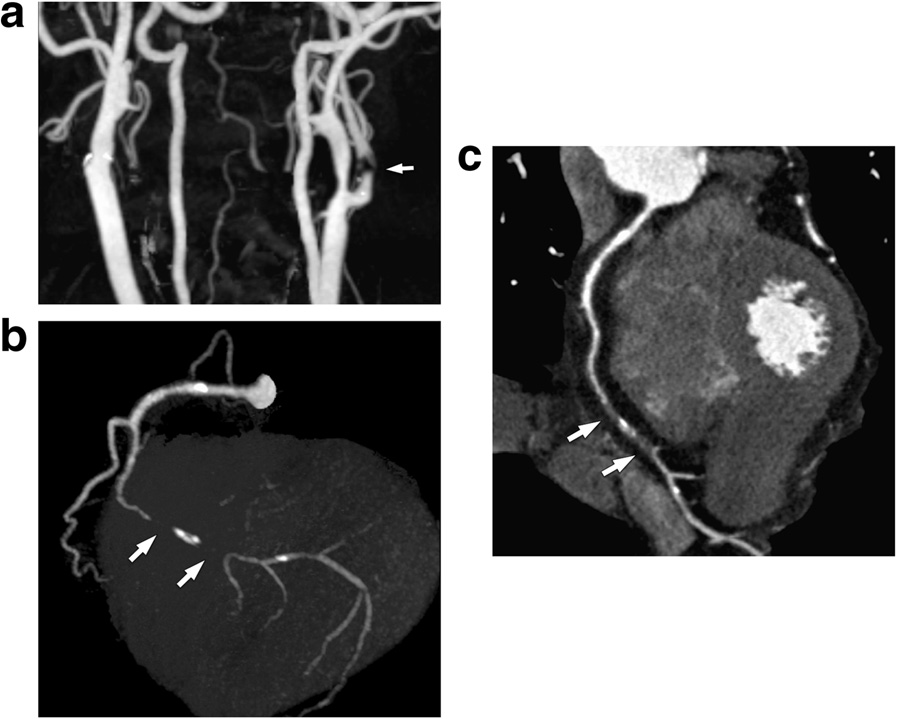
Carotid CT- (a) and coronary CT angiograms (b and c) of a 70-year-old asymptomatic male undergoing carotid endarterectomy. Carotid CT angiogram (a) shows severe stenosis of the left internal carotid artery. For screening of coexisting coronary artery disease, he underwent coronary CT angiogram prior to carotid endarterectomy. Coronary CT angiographic images (b): maximum intensity projection image [angiographic view], (c): curved planar reformation image) show a long mixed plaque with severe luminal stenosis in the distal right coronary artery.
